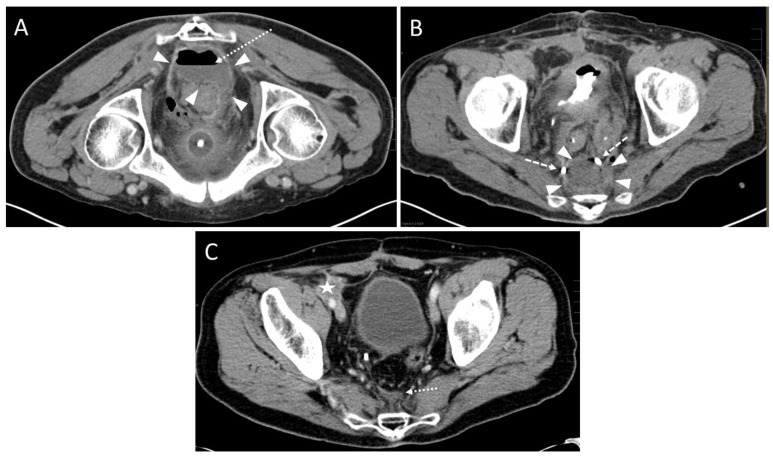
A 67-year-old male with a history of rectal carcinoma (T4 tumor stage) after deep anterior rectal resection including total mesorectal excision and the creation of a loop-iliostomy. In addition, a ureter reimplantation due to tumor infiltration in the bladder and an iliac lymph node dissection was performed. Five days after the operation a small anastomotic insufficiency was diagnosed and treated by flushing. Three weeks later a CT fluoroscopy-guided drain placement was performed due to recurrent fever spikes up to 38.5 degrees. (A) A preinterventional scan showed a presacral fluid collection (arrowheads) with air/fluid level (dotted arrow). (B) After the CT fluoroscopy-guided insertion of an 8 F drain, purulent fluid could be partially aspirated and a postinterventional control scan showed a moderate reduction of the abscess formation (arrowheads). Dashed arrow: loop-formation of the distal drain segment. (C) After one year, the complete resolution of presacral abscess formation was observed. Dotted arrow: small scar tissue. However, a newly appeared soft tissue mass in terms of a peritoneal tumor relapse occurred in the right parailiac region (asterisk).
